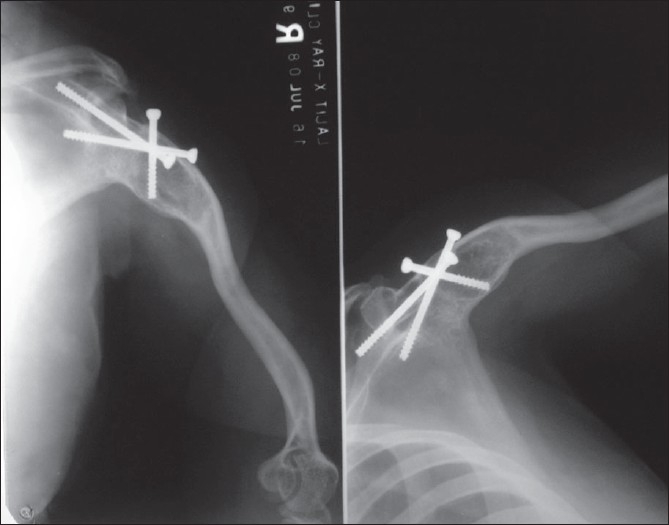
Radiographs (anteroposterior and lateral view) humerus at 10 years showing arthrodesed shoulder and length of humerus achieved
